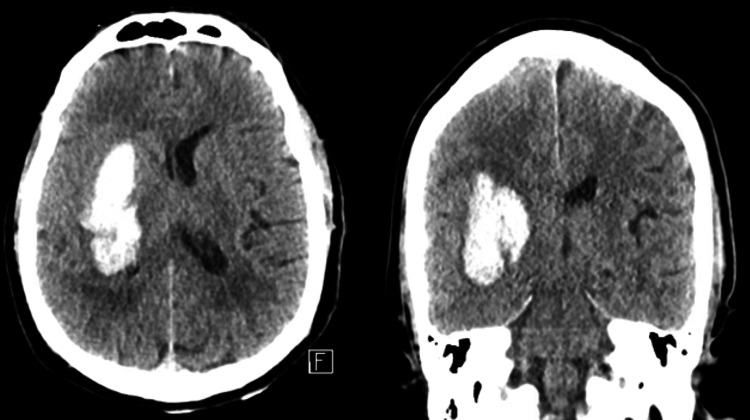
Pre-operative CT scan for patient 3 CT scan showing a large right basal ganglia ICH ICH: Intracerebral hemorrhage
